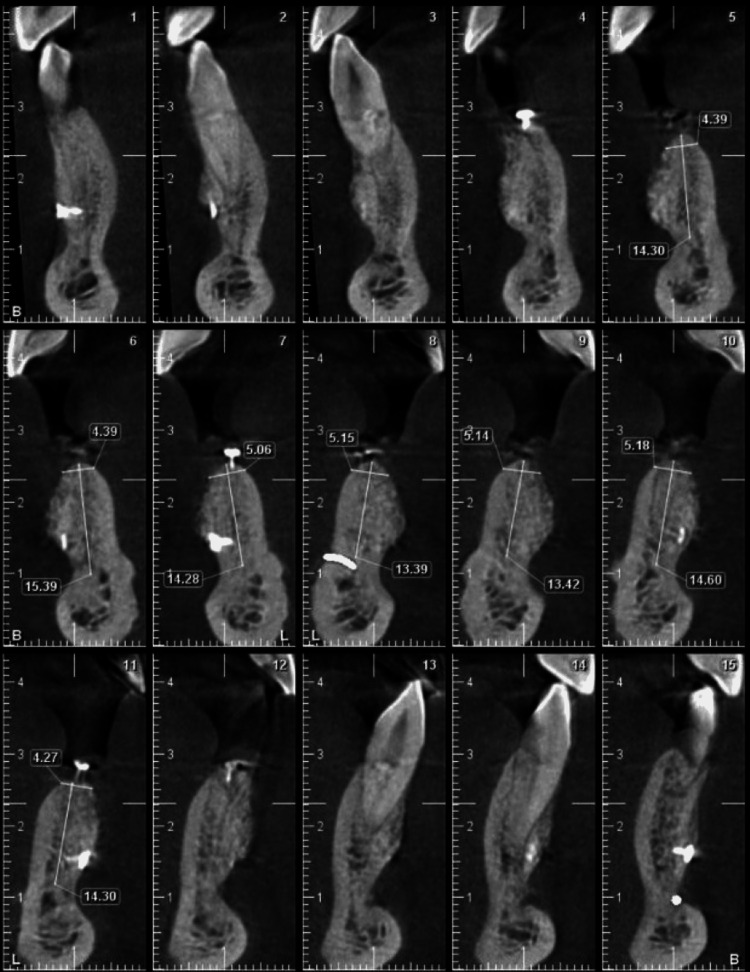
Postoperative CBCT of the anterior mandible showing the regenerated site. CBCT, cone beam computed tomography
